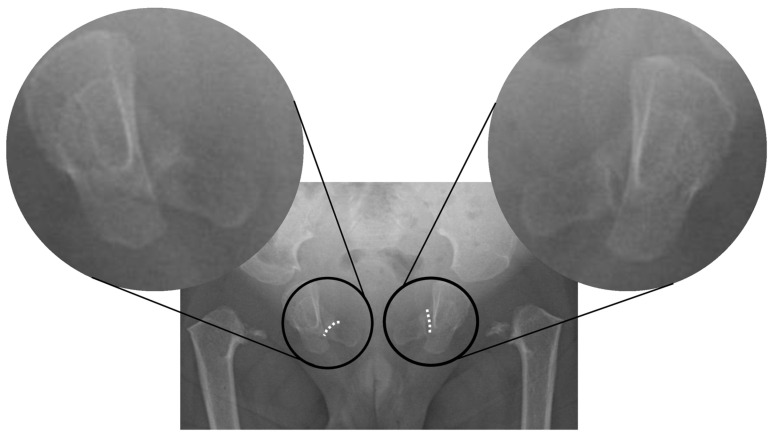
Anteroposterior pelvic radiograph of a 2.5-year-old patient with SEDC showing multiple ossification centers as a vertical radiolucent line dividing the ramus into medial and lateral portions (white broken lines).
